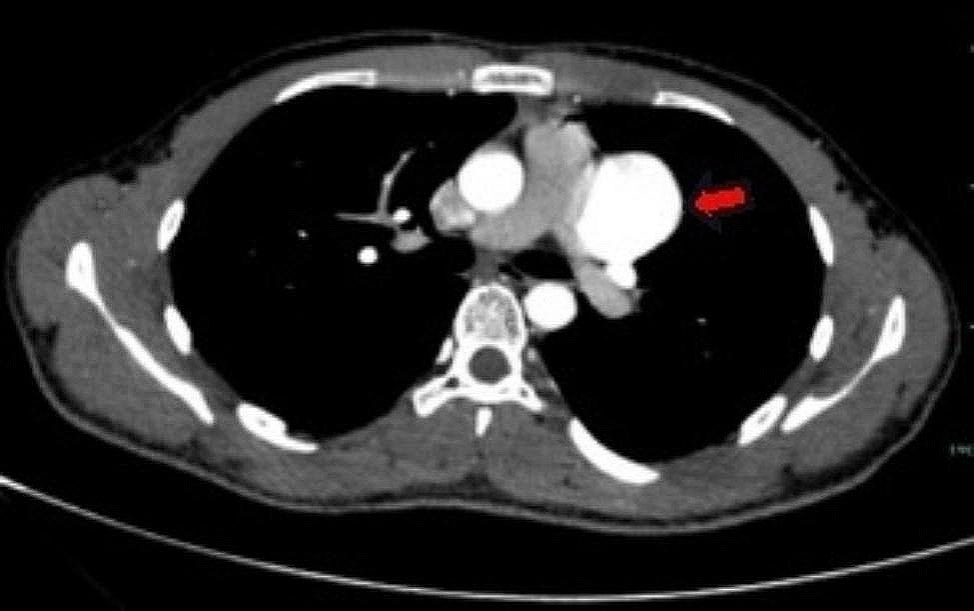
Computed tomography angiography of pulmonary vein
